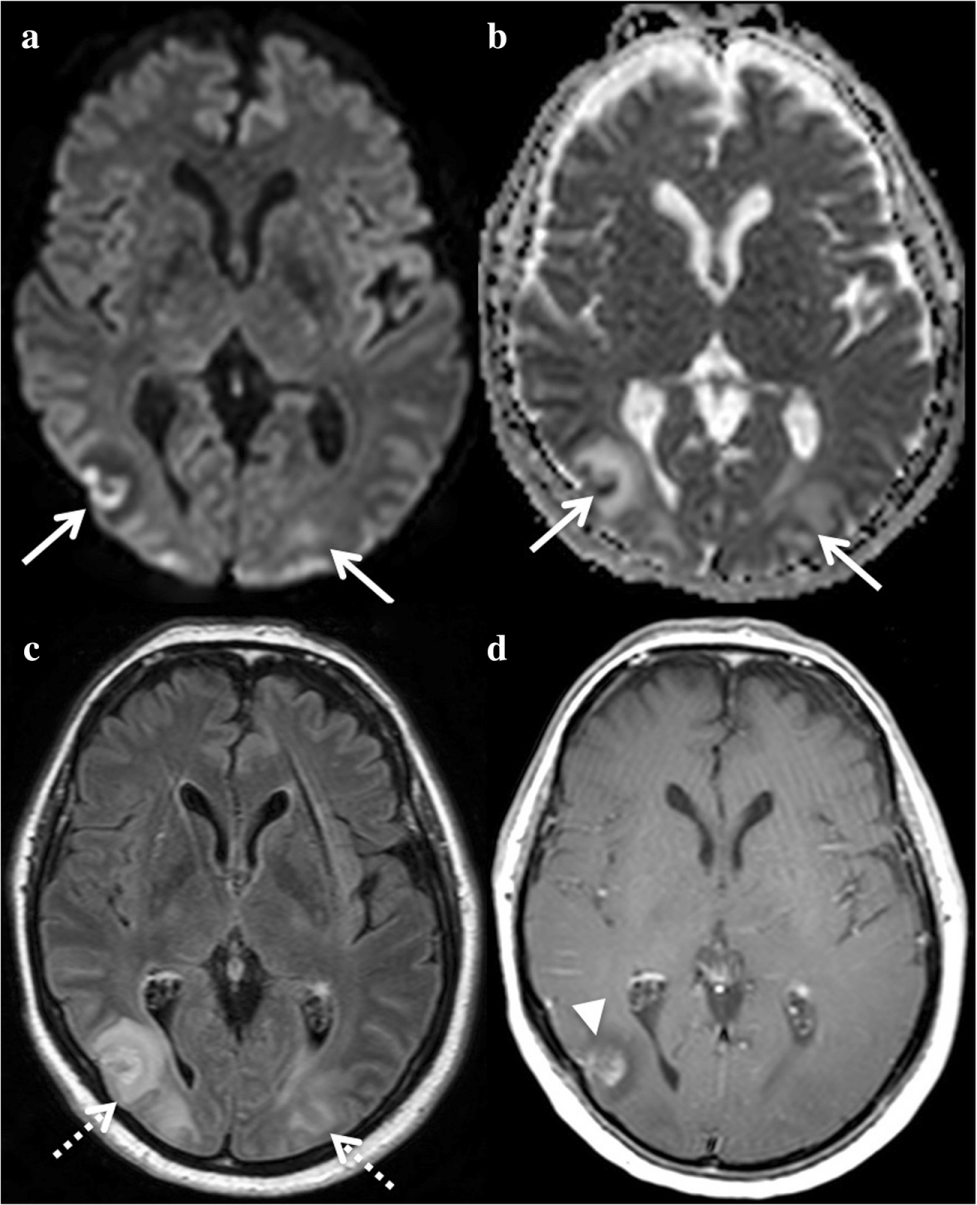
A 40-year-old woman with posterior reversible encephalopathy syndrome (PRES) presenting with postpartum attention deficit disorder. DWI shows both vasogenic and cytotoxic oedema with increased and decreased ADC (a, b, arrows). Posterior lesions appear as hyperintensities on FLAIR (c, dotted arrows). Contrast-enhanced T1-WI shows cortical enhancement of one lesion (d, arrowhead)
